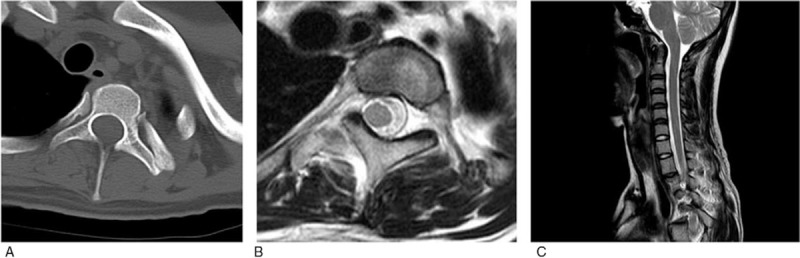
(A) CT axial Th2 spine level; (B) MRI T2WI axial Th2 spine level; (C) MRI T2WI sagittal. CT = computerized tomography, MRI = magnetic resonance imaging.
